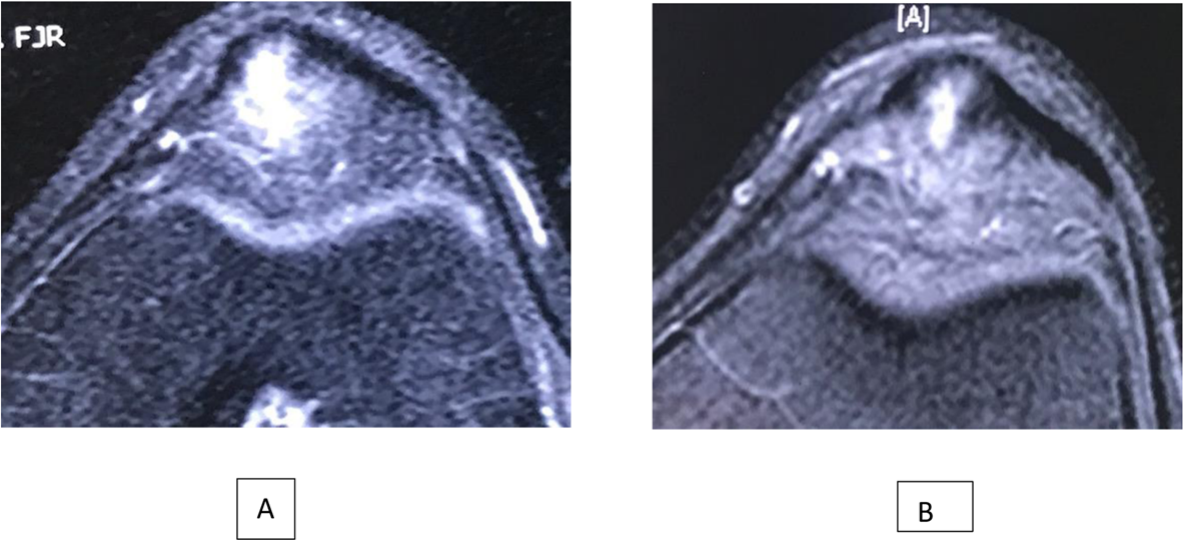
A: Axial STIR MR image of the tear of the patellar tendon pre-injections or baseline. B: MRI axial view of the patellar tendon at 6 months post-injections
